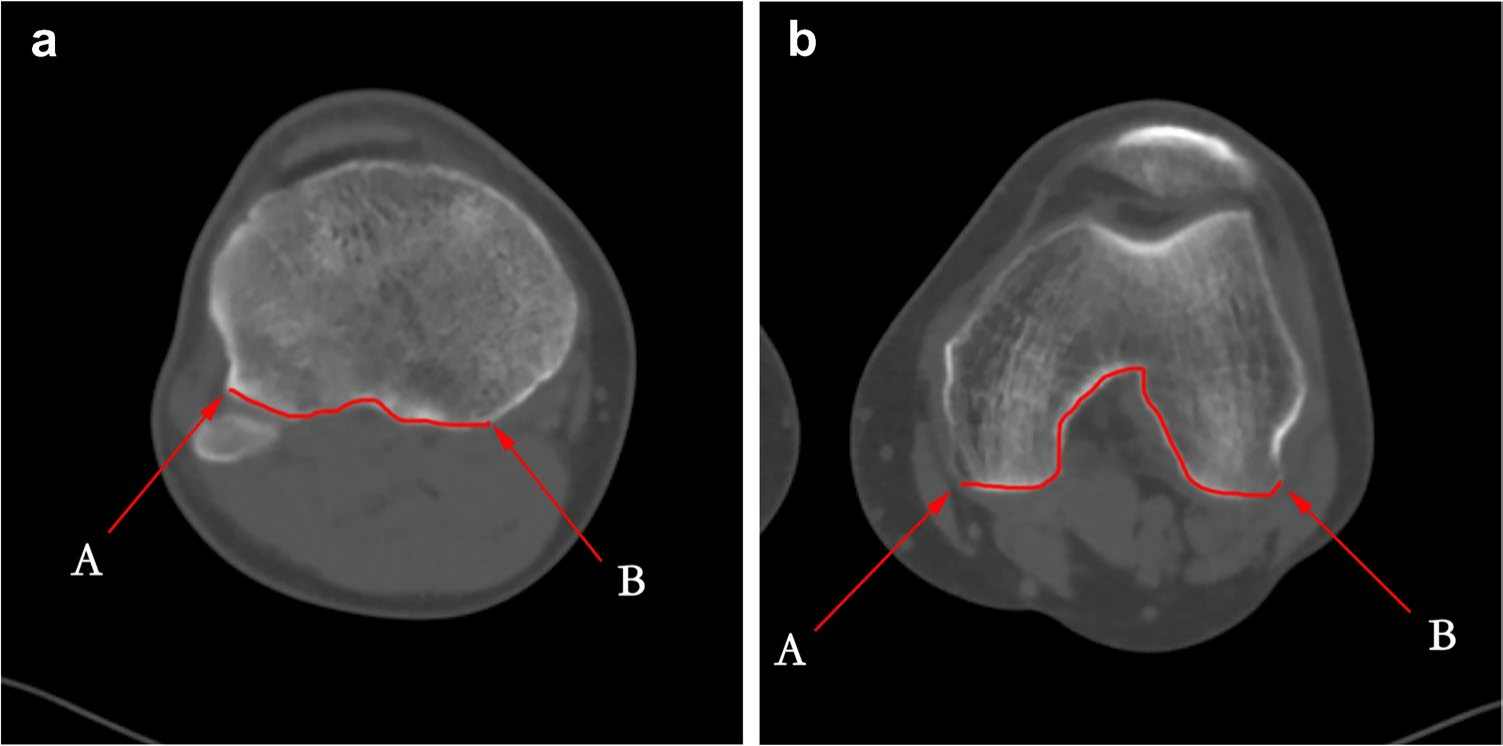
Transverse CT images of the knee joint with superimposed measurement boundaries (red). a Proximal tibia and fibular head showing the anterior-most point of the fibular head (arrow A, end of line segment) and the posteromedial ridge of the proximal tibia (arrow B, end of the line segment). The red superimposed line represents the posterior boundary of the posterior cortex of the proximal tibia. b Posterolateral (arrow A, end of the line segment) and posteromedial (arrow B, end of the line segment) parts of the femoral condyle. The red superimposed line represents the posterior boundary of the posterior cortex of the distal femur
